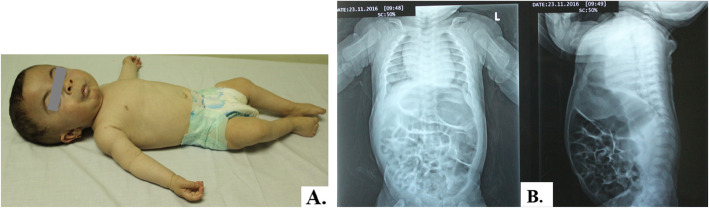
Patient no. 6 at the age of 1 year. A Skeletal X-rays showing short humerus, horizontal oar-shaped ribs. B Lateral X-ray spine showing short biconvex vertebral bodies and oar-shaped ribs. Note high square forehead, short upturned nares, chest deformity, and short limbs
